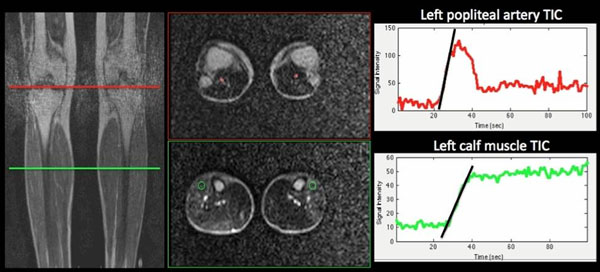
Analysis of FP MRI images
